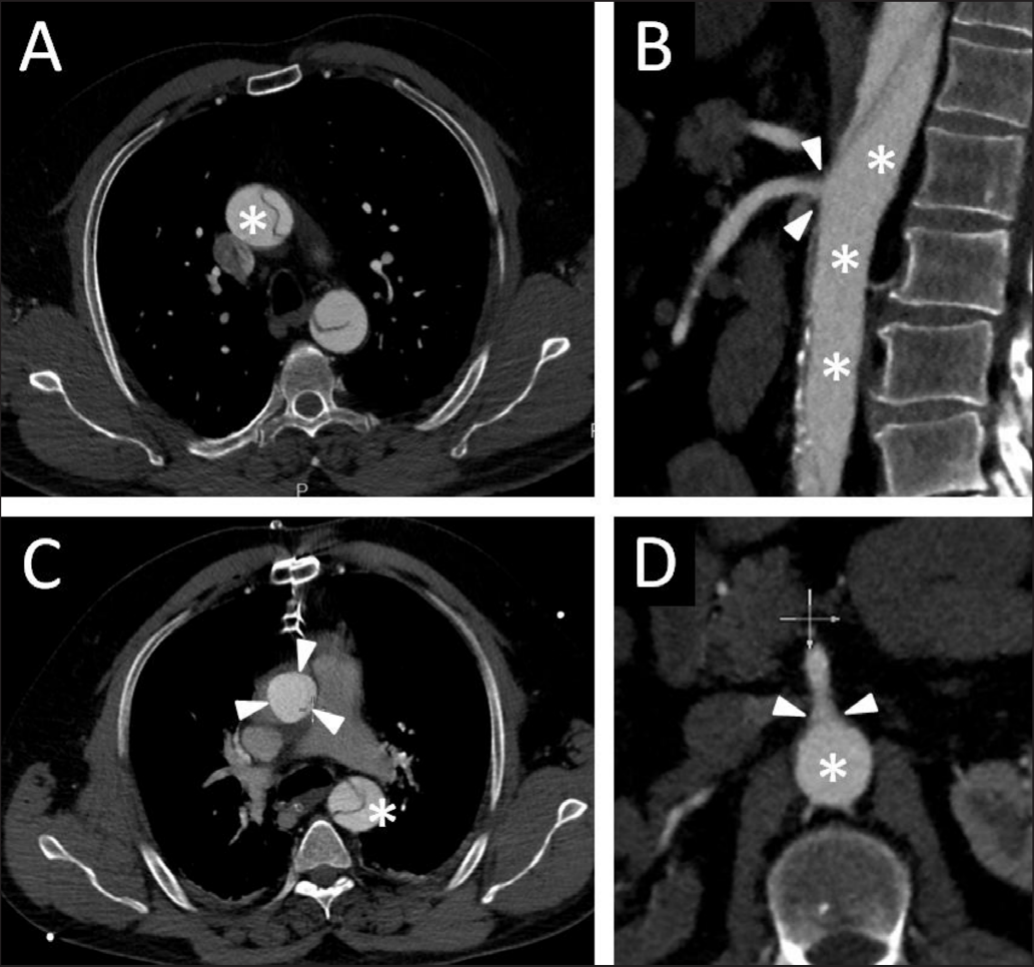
CT angiography of the aorta shows aortic dissection before (a) and after repair (b). The ostium of the superior mesenteric artery (c, d, arrowheads) is supplied from the false lumen (asterisks).
